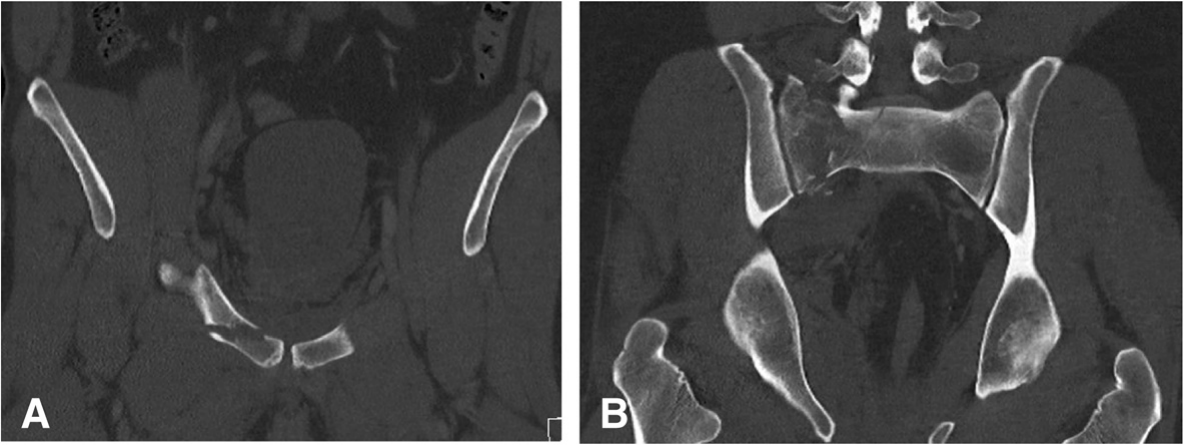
Initial computed tomography scans. Pelvic computed tomography with SAM Pelvic Sling™ II in place. Fracture of the right superior pubic ramus with regular configuration of the pubic symphysis (a). Ipsilateral fracture of the sacrum (b)
